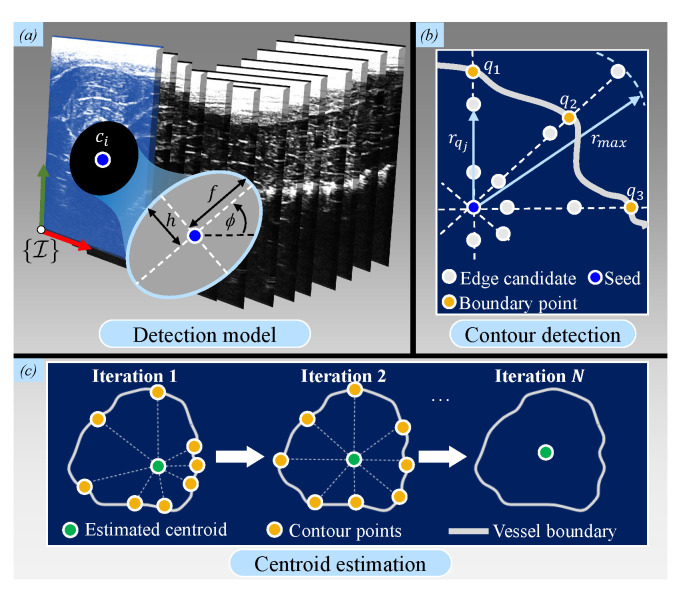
Ultrasound (US) image arterial reconstruction scheme. (a) For each US image slice, the arterial contours are extracted and reconstructed centroids ($c_{i}$) are estimated. Knowledge of the ellipse parameters (*f*, *h*, and ϕ) are required and included in an extended Kalman filter; (b) equi-spaced edge candidates along a radial line with maximum predefined length ($r_{max}$). For each candidate (${}_{j}$) in line (*q*), the distance to the center along this line is defined as $r_{q_{j}},j\in N\;\left(j=1,\ldots ,N\right)$. (c) Each centroid of the US slices is estimated at each iteration (*q*), repeated iteratively, until the calculated center converges to the weighted center.
